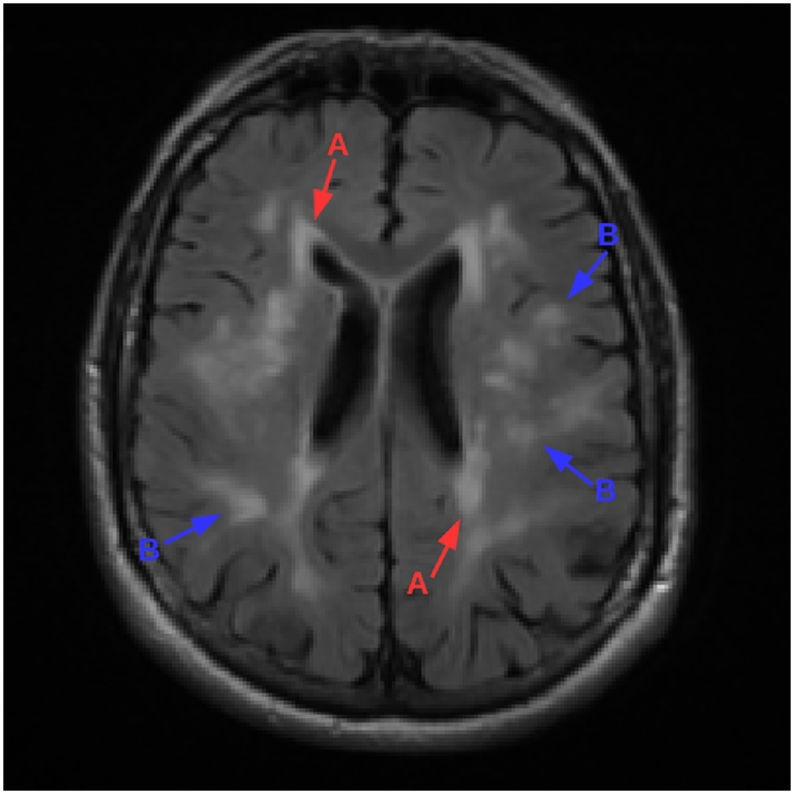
T*_1_-w (left) and FLAIR (right) image of a subject with periventricular (labeled A) and deep (labeled B) white matter lesions. Note that pathology is more visible on the FLAIR image than it is on the *T*_1_-w image.
